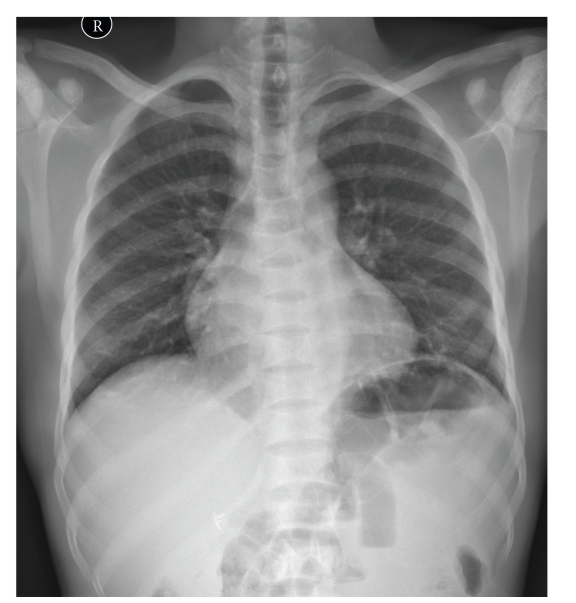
Chest radiograph showing coarsening of trabecular pattern with loss of corticomedullary differentiation of the ribs subject to haematopoietic marrow replacement in a patient with sickle cell disease. Note avascular necrosis involving the humeral heads bilaterally and colonic gas replacing splenic shadow in the left upper quadrant (secondary sign of autosplenectomy/small spleen subject to previous infarcts).
